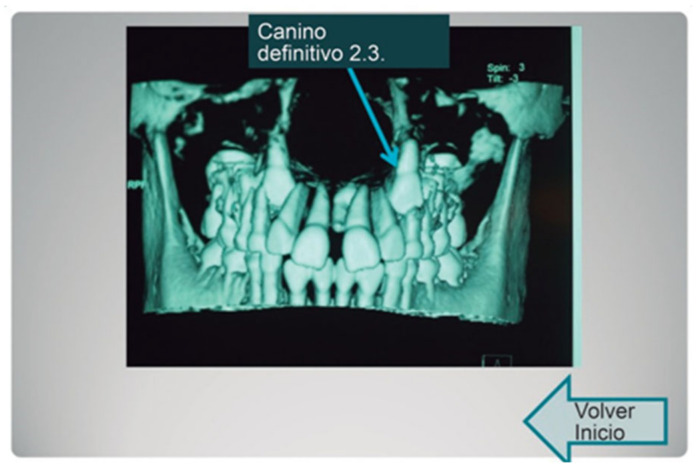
Example of the CT image chosen for the clinical cases.
